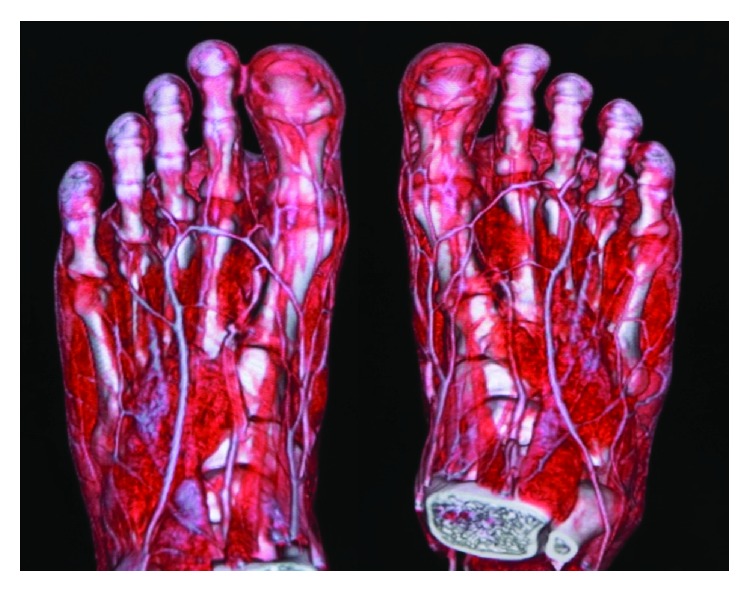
Enhanced computed tomography was used to evaluate toe vasculature before surgery.
